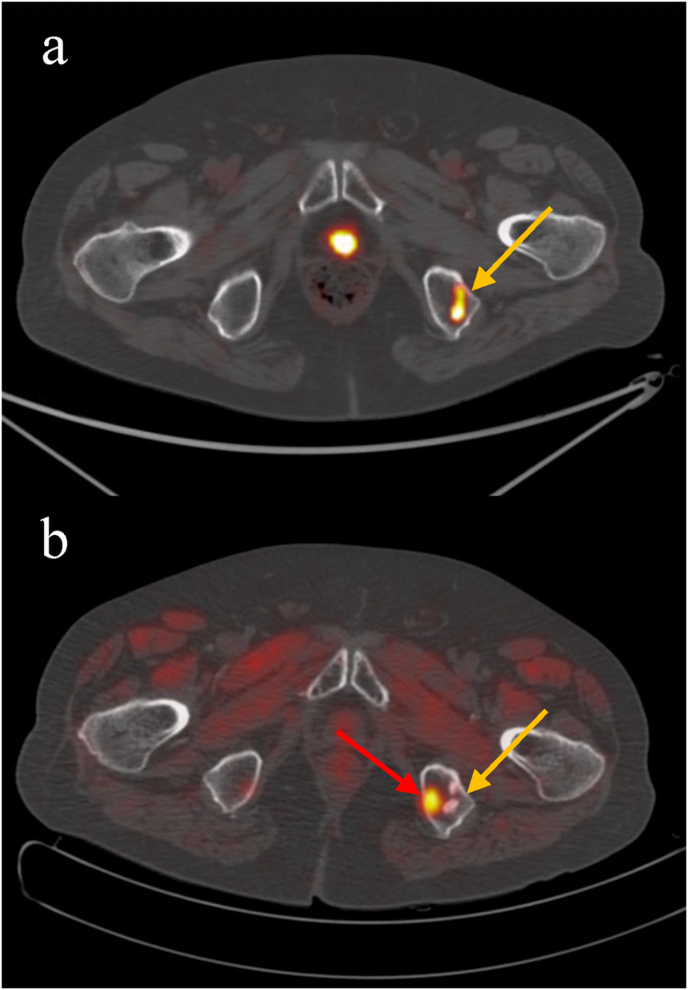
a) Pre-RLT axial fused [^68^Ga]Ga-PSMA-11 PET/CT showing intense uptake in sclerotic lesions of the left ischiatic bone (orange arrow); b) post-RLT axial fused [^18^F]Choline PET/CT showing faint uptake in the known sclerotic lesions of the left ischiatic bone (orange arrow) associated with the presence of a focal and intense medullary localizations (red arrow). (For interpretation of the references to colour in this figure legend, the reader is referred to the Web version of this article.)
